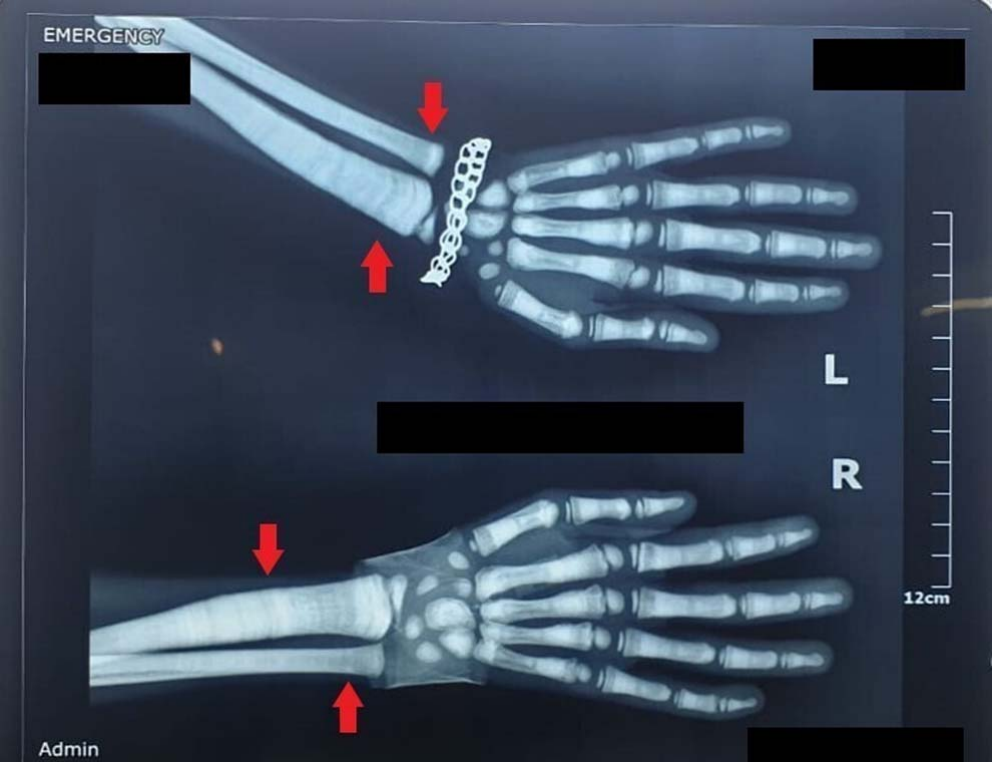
Radiographs of the hands show alternating bands of radiodense and radiolucent lines at the distal metaphyses of the radii and ulnae (arrows).
